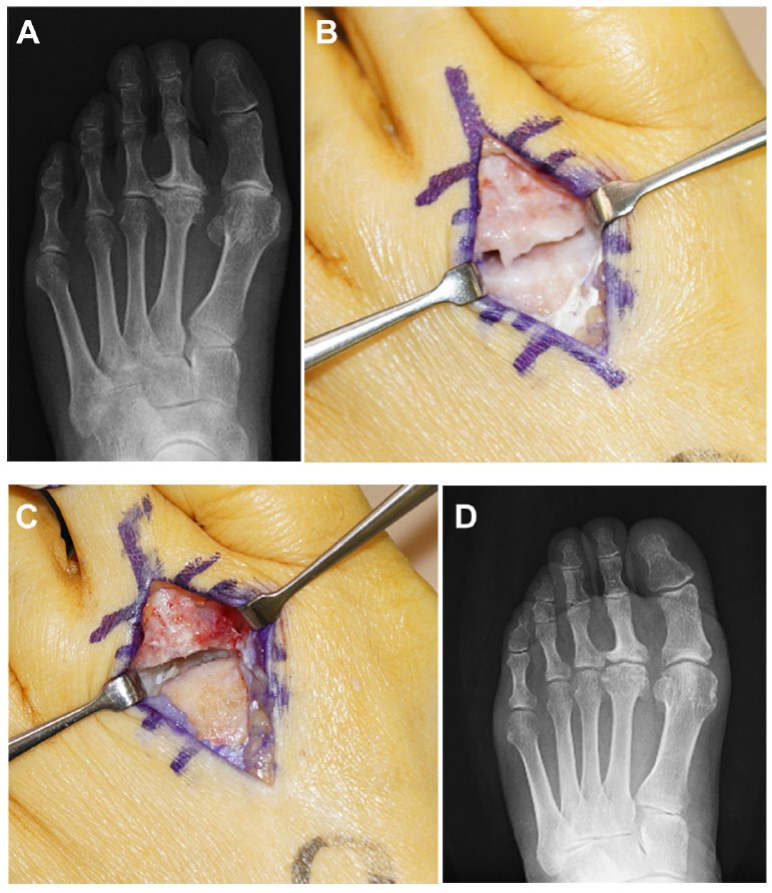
Open debridement. (A) Osteoarthritis in second metatarsophalangeal (MTP) joint by Freiberg disease. (B) Preoperative radiograph. Joint space narrowing and osteophyte formation were observed in the second MTP joint. (C) After removal of the osteophyte and synovium. (D) Postoperative radiograph. Widening of the MTP joint were observed after debridement.
